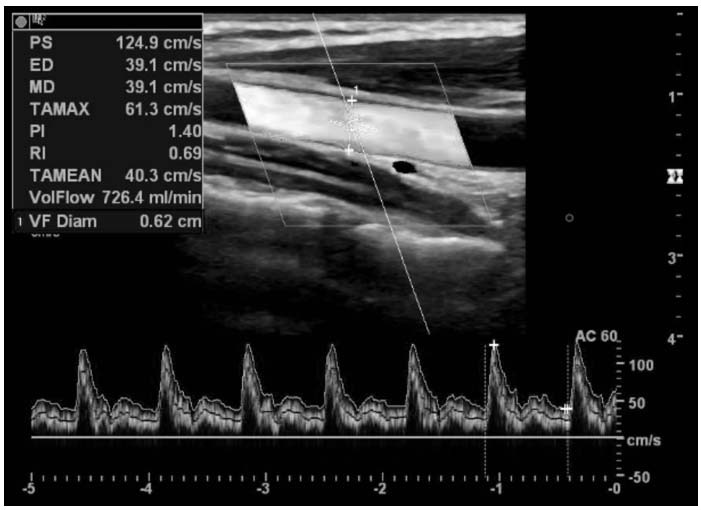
Left CCA duplex ultrasonography image, showing PS, ED, PI, RI, vessel diameter, and flow volume values during the premenstrual headache period.
